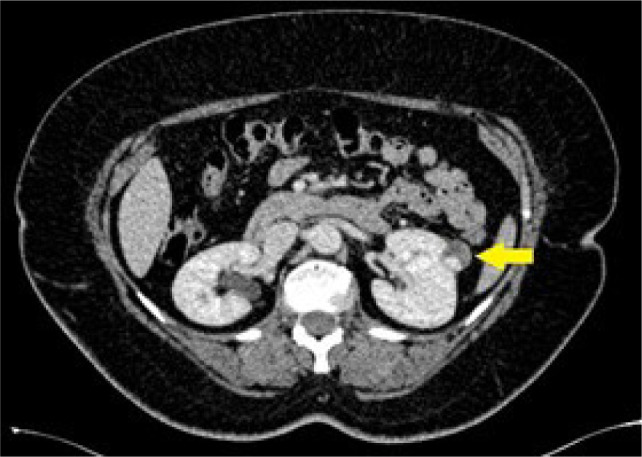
Axial CT at the level of renal hila in a 54-year-old female patient showing a partially solid mass lesion in the left kidney with enhancing small soft tissue component (arrow) and evident angular interface sign. The mass was resected and pathologically showed clear-cell renal cell carcinoma.
